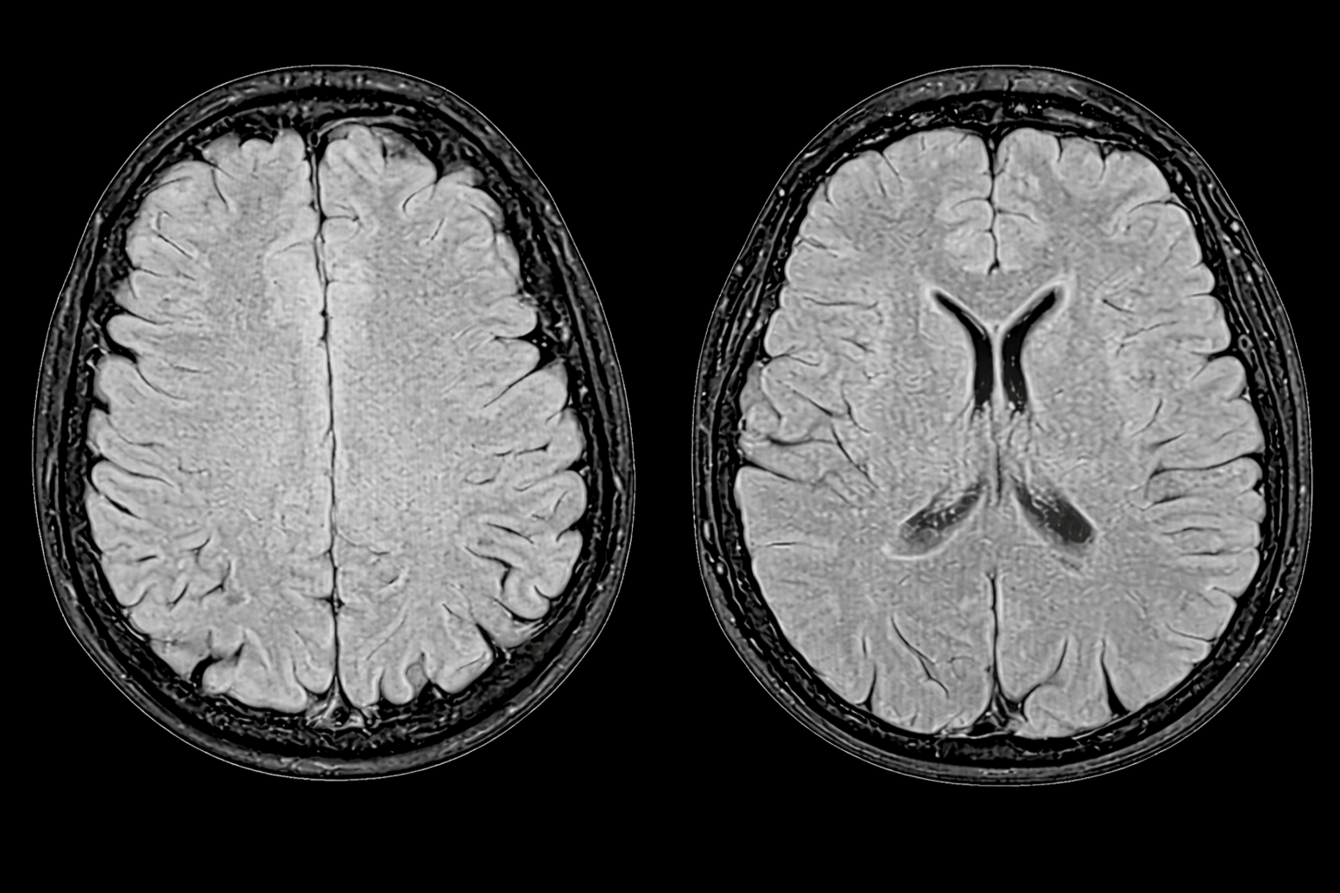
Magnetic Resonance Imaging (MRI) of the Brain Normal Brain Parenchyma and Ventricular System; No Focal Structural Abnormality Was Noted.
